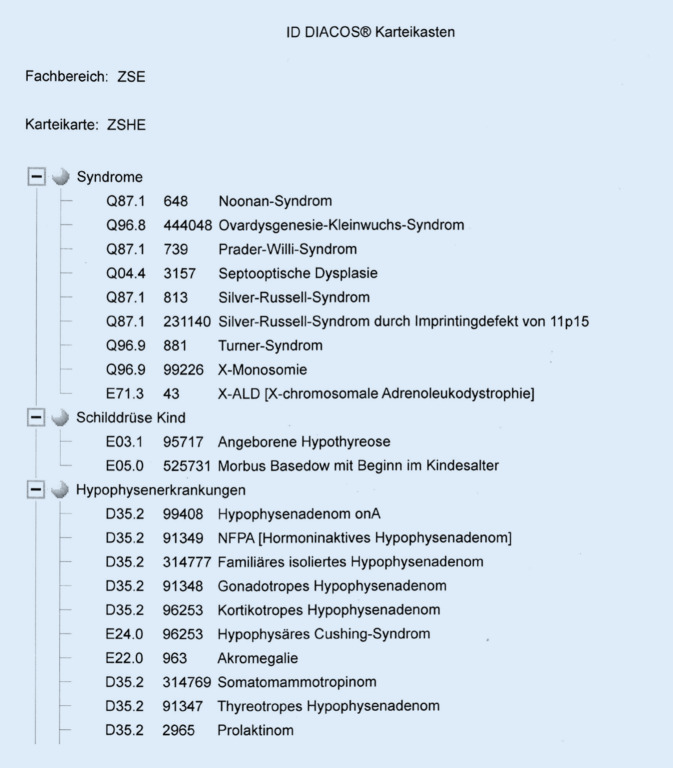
Seit 2020 hat die Anzahl der Orpha-kodierten Patient*innen Auswirkung auf die Finanzierung des jeweiligen ZSE-Fachzentrums, wodurch auch ein finanzieller Anreiz für die Orpha-Kodierung geschaffen wurde. Basierend auf dem Würzburger Parametrisierten Dokument (Abb. 3) soll zudem zeitnah eine weitere Maske zur Dokumentation am ZSE Tübingen implementiert werden, welche alle Elemente des „Set of common data elements for Rare Diseases Registration“ [14] der Europäischen Plattform für die Registrierung Seltener Erkrankungen abdeckt. Unter anderem kann dann die Symptomatik anhand von HPO erfasst werden. Eine Kodierung via SNOMED CT ist bisher nicht vorgesehen.
